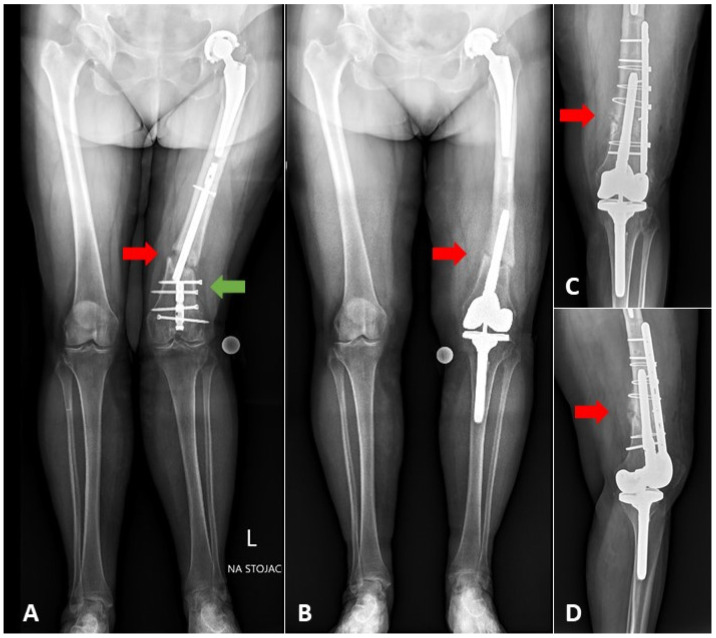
(A) AP long-standing radiograph of the patient with the fracture of 1/3 distal part of the femoral shaft, with the broken retrograde femoral nail (green arrow), and knee osteoarthritis; (B) long-standing radiograph performed nine months after the one-stage TKA with long-stem extension. Bone union was not achieved due to insufficient stability of fracture causing excessively short (64 mm from the tip of stem to fracture level) and undersized stem (13 mm stem diameter filling 68% of medullary canal in the narrowest point). Shorter femoral stem was used due to the presence of hip prosthesis ipsilaterally. (C) AP and (D) lateral radiographs after re-stabilization with the additional plate fixation. Red arrows indicate fracture level.
